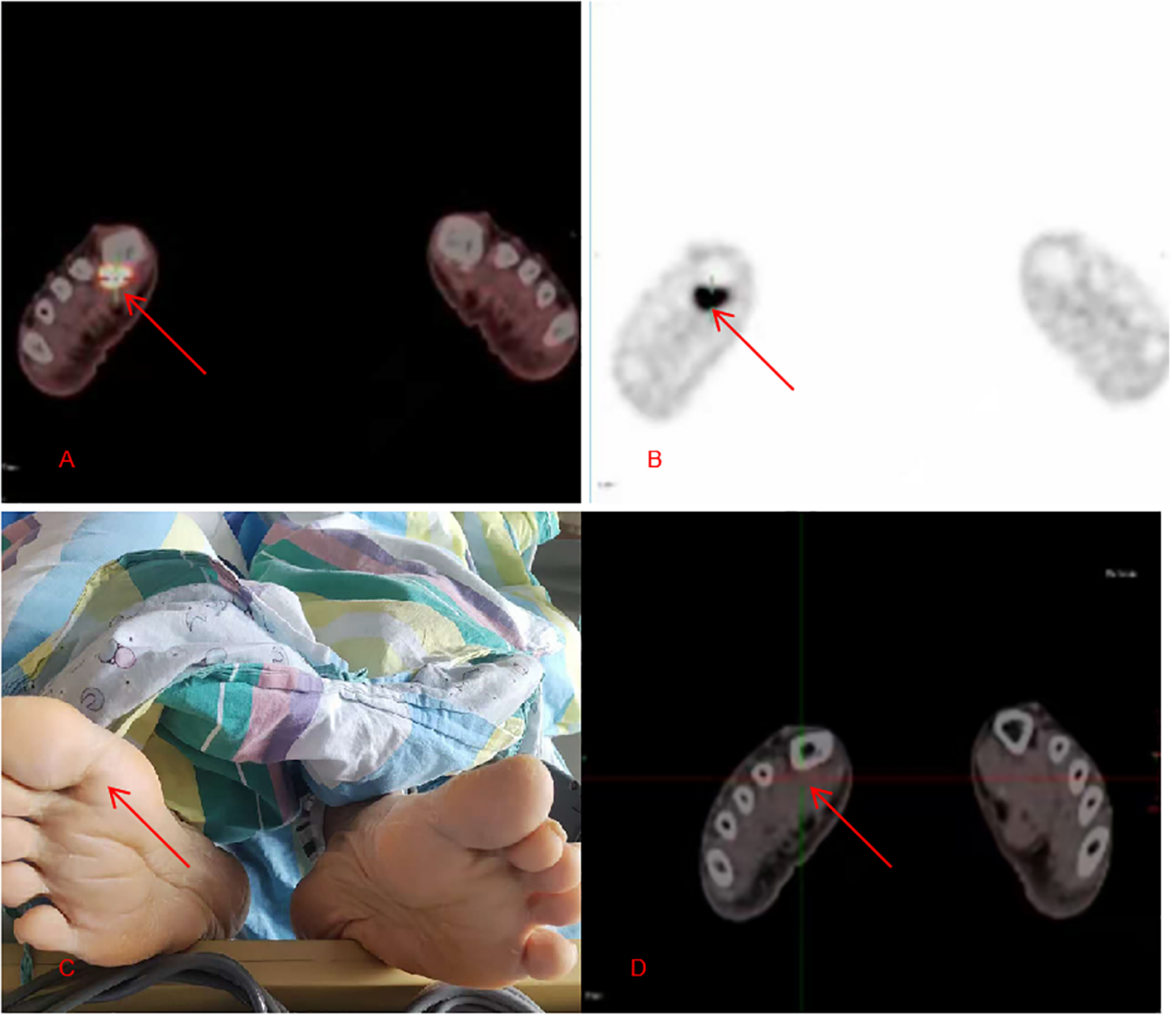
(A, B) (Pre-treatment): PET-CT shows an FOG-avid lesion at the right metatarsophalangeal joint (red arrow); (C) (Pre-treatment physical examination): No cutaneous pigmentation is observed at the right plantar metatarsophalangeal joint (red arrow); (D) (Post-treatment): PET-CT shows disappearance of the previously observed FOG-avid lesion at the right metatarsophalangeal joint (red arrow at the intersection of the red and green lines).
